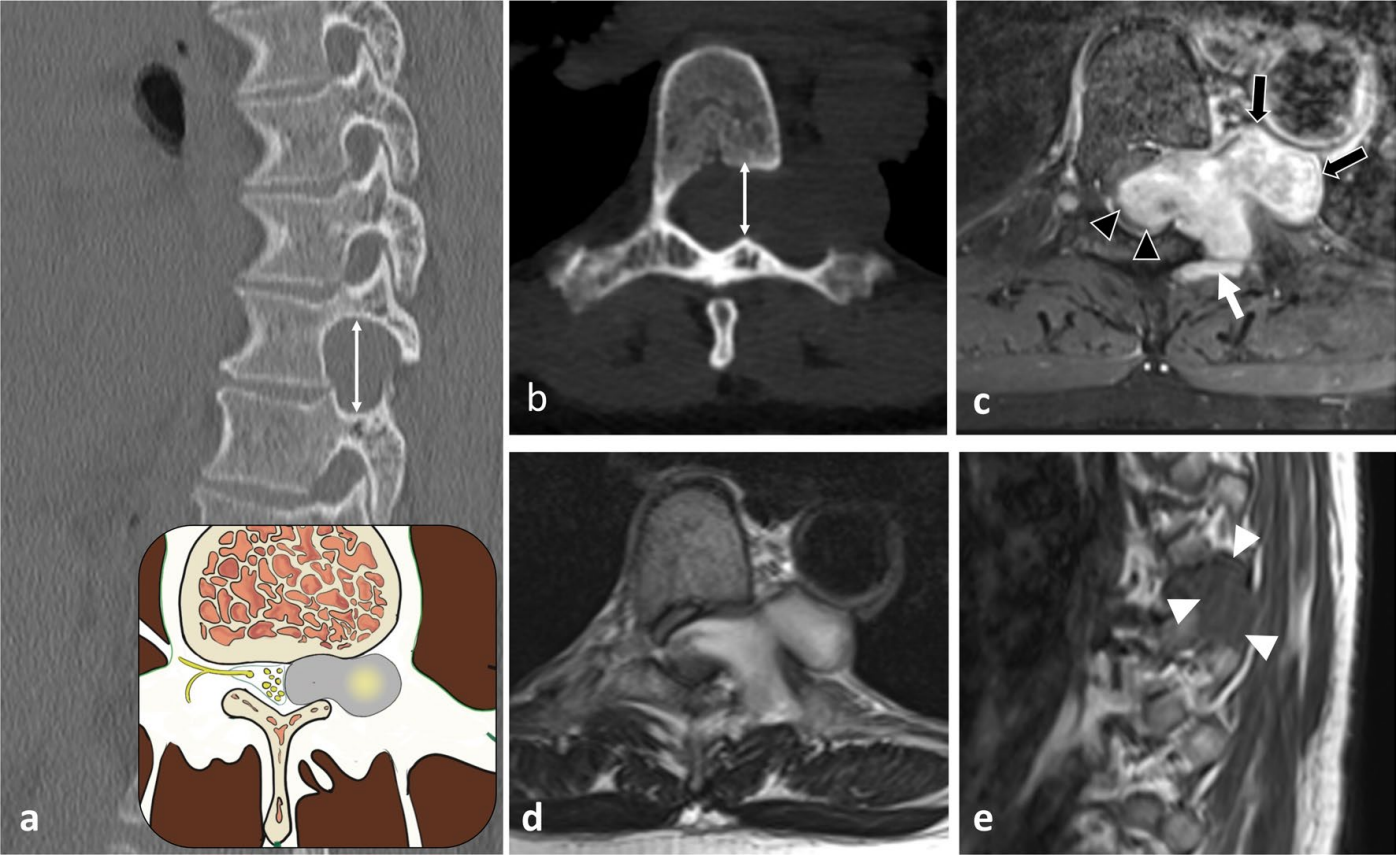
Neurofibroma* of the 7th left spinal nerve in a 36-year-old female with thoracic radicular pain. Sagittal (a) and axial CT reconstruction (b) shows the widening of neural foramen (double arrows). Axial CT (b), axial T1WI with contrast (c) and axial T2WI (d) demonstrate the typical dumbbell configuration of nervous tumour with intraspinal (back arrowheads) and paraspinal components (black arrows) that communicate via the intravertebral foramen. The mass presents high signal intensity on T2WI (d), low signal intensity on T1WI (e) and homogeneous enhancement (d). The nervous mass extends into the multifidus muscle (white arrow) and the intercostal space (white arrowheads). The yellow star shows the epicentre of the mass
